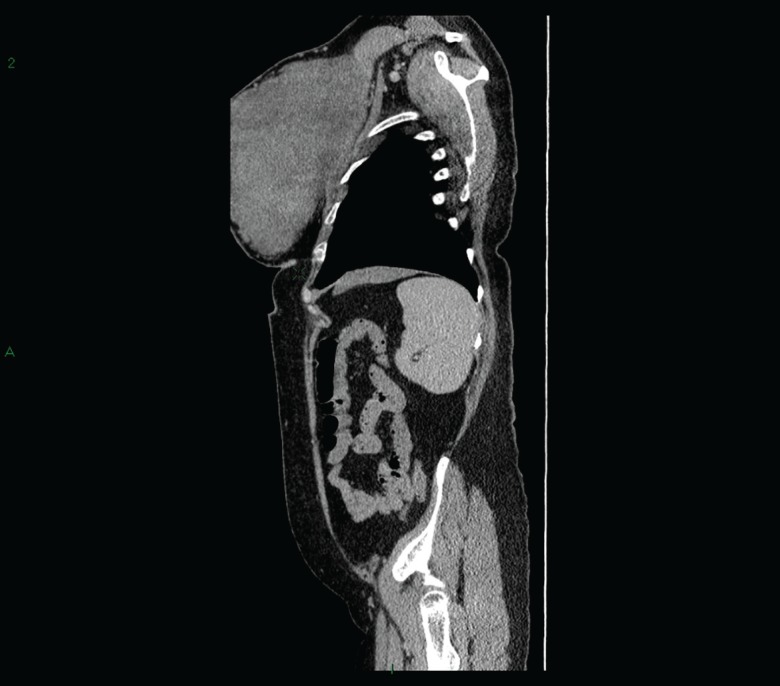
CT revealed a large solid heterogeneous left breast mass going off the CT window measuring at least 20 cm in a single oblique measurement
